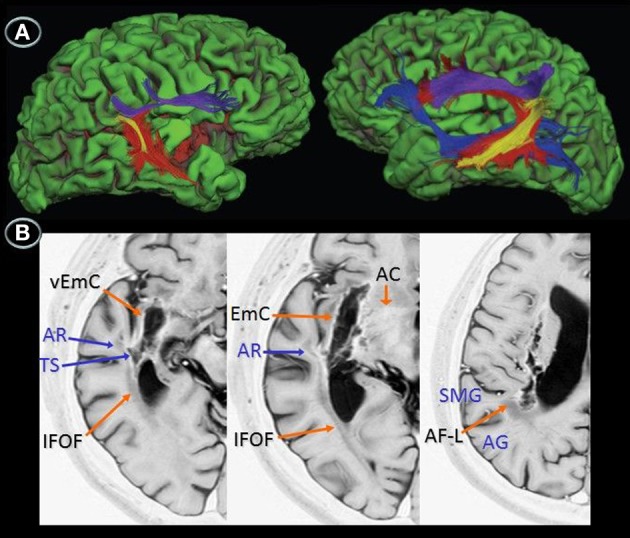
Diffusion tensor imaging (3T MRI) of patient JAM. (A) Uninflated surface of the cerebral hemispheres (FreeSurfer reconstruction) depicting gyri in green and sulci in red. The right image shows a small cortical component of the haemorrhage (red) involving the right anterior insula and superior temporal gyrus. The diffusion tensor imaging reconstruction of the arcuate fasciculus and inferior fronto-occipital fasciculus shows (left image) damage to the right long direct segment of the arcuate fasciculus (red) and inferior fronto-occipital fasciculus (blue) with relative sparing of short and long fibers of the anterior indirect segment (purple) and posterior segments (yellow), whereas the right image shows fully developed left perisylvian white matter pathways. (B) Anatomical axial MRI section (Short T1 Inversion Recovery—STIR—sequence) show the right striatocapsular lesion and perinecrotic tissue with degeneration of several white matter tracts (orange and blue arrows). AR indicates, auditory radiations; TS, temporal stem; SMG, supramarginal gyrus; AG, angular gyrus; EmC, extreme capsulae; vEmC, extreme capsulae; IFOF, inferior fronto-occipital fasciculus; AC, anterior commissure; AF-L, arcuate fasciculus-long segment.
